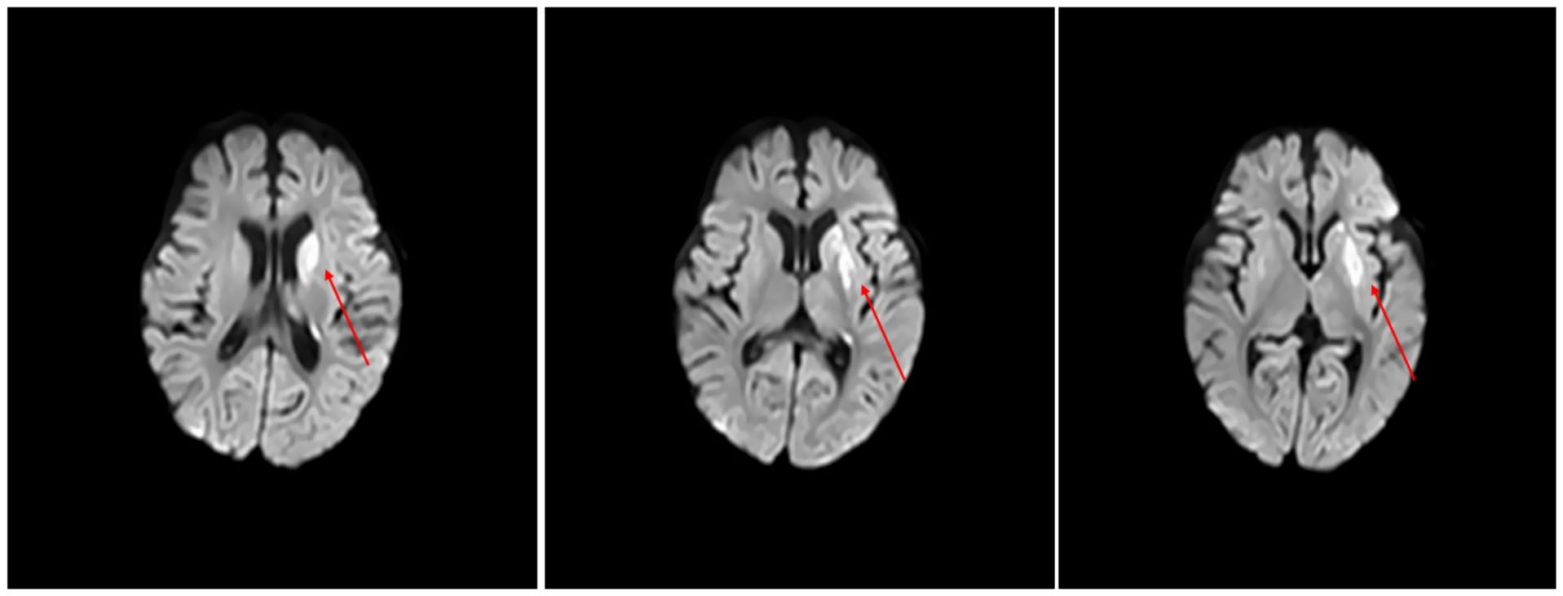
A brain MRI of the patient showing a markedly hyperintense lesion in the left cerebral hemisphere on DWI, indicating restricted diffusion consistent with infarction-like lesion.
